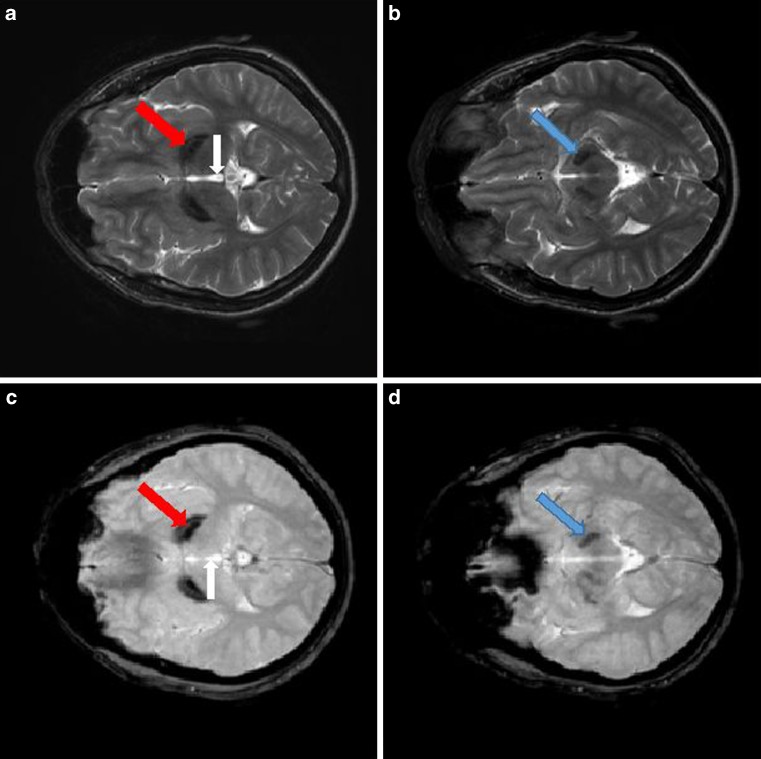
Magnetic resonance imaging results from MPAN patient. a and b T2-weighted images, c and d T2*-weighted images. The hypointense signal in globus pallidus (a and c) and substantia nigra (b and d) is due to iron accumulation. The third ventricle is indicated by a *vertical arrow*. Notice a medial medullary lamina between the internal and external part of the GP in T2-weighted images – a radiological phenomenon typical for MPAN patients (*red arrow*). Hypointense signal in SN is often less prominent than in GP (*blue arrow*)
